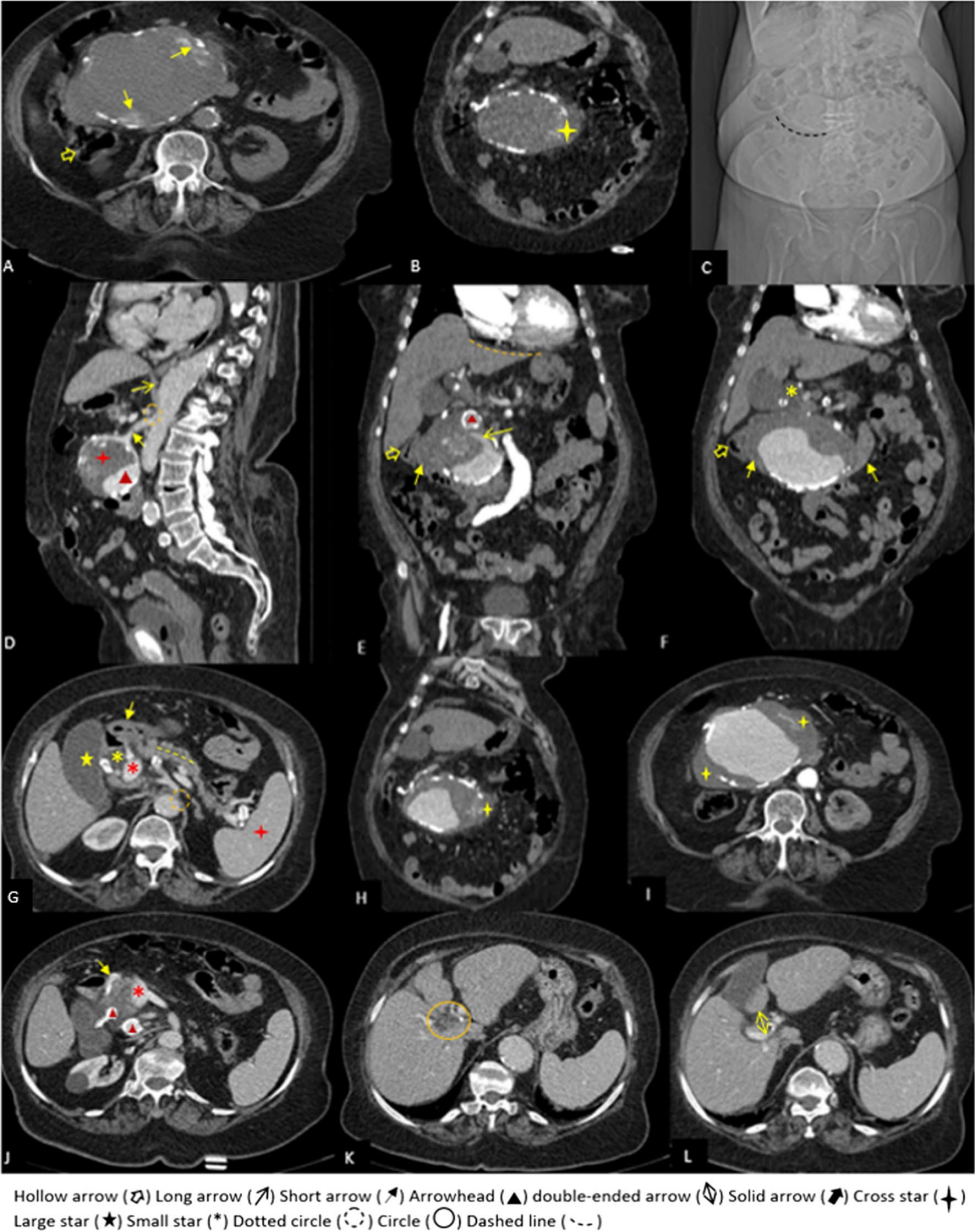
A, B non-contrast axial and coronal computed tomography scan show a huge hetero-dense mass in the sub-hepatic region, near to the head of the pancreas and medial to the cecum (yellow hollow arrow), with focal bulging at its medial margin (yellow cross star) and peripheral calcification and marginal hyper-dense crescent-like foci (short yellow arrows) suggestive of probably an aneurysm with hyperdense crescent sign. C Abdominal computed tomography scan Topogram: curvilinear calcification is visible mainly at right side of thoracolumbar spine in the sub-hepatic region. D Sagittal plane of computed tomography angiography, reveals an aneurysm with mural thrombosis (red cross star) and its patent lumen (red arrowhead) originating from the posterior and inferior surface of the proximal of the superior mesenteric artery (short arrow). The origin of the celiac trunk (dotted circle) is significantly narrowed due to the compressive effect of thickened diaphragmatic crura (long arrow). E, F Coronal plane of computed tomography angiography shows considerable compressive effect of huge aneurysm on the cecum, D2 and D3 portions of the duodenum (short yellow arrows) and common bile duct (small yellow star). A thrombus fissuration sign (long yellow arrow) is an extension of contrast from the patent lumen to the mural thrombus. The red arrowhead points to the another aneurysm, located at the superior margin of the giant aneurysm. The orange dashed line shows the slightly irregular liver’s margin. G Portal vein (small red star) and dilated common bile duct and are visible on the axial plane in the porta-hepatis region. The gallbladder is shown by the large star. The pylorus and D1 portion of the duodenum are indicated by the small yellow arrow. A yellow dashed line represents mild dilatation of the main pancreatic duct. The celiac trunk's origin has narrowed significantly (dotted circle). The red cross star represents the spleen. H, I The yellow cross stars indicate focal bulging and discontinuous calcification at the medial and lateral margins of the huge aneurysm. Mild fat stranding is present, adjacent to medial margin of the aneurysm. J The axial scan just inferior to "G". Gastroduodenal artery (GDA) is shown by short yellow arrow. Two aneurysms originating from the posterior branch of GDA (posterior superior pancreaticoduodenal artery) are depicted by the red arrowheads. (K, L) The central intrahepatic bile ducts are mildly dilated. The anterior periportal space is widened
